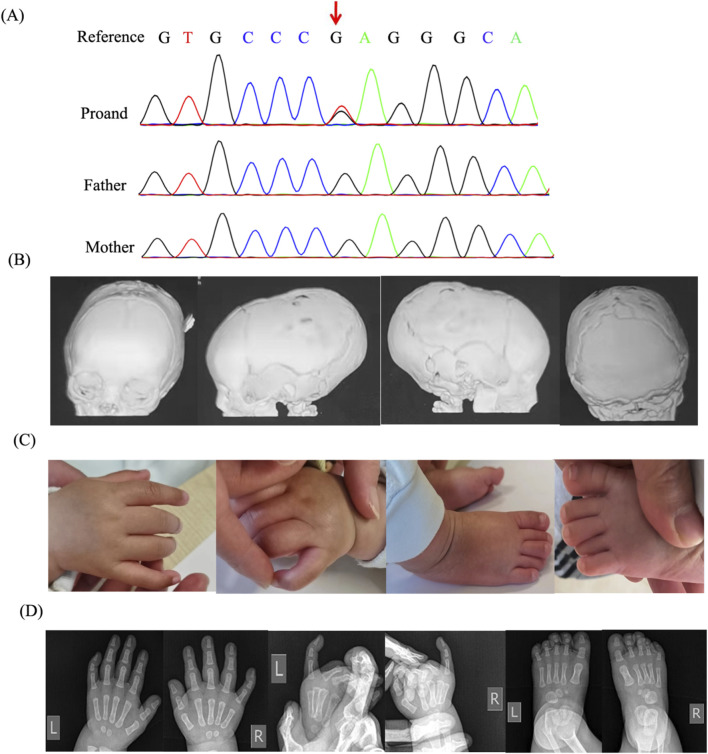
Clinical and genetic features of the proband (P11) harboring the *NOG* variant (c.562G>T, p. Glu188Ter). (A) Sanger sequencing confirming the *de novo* variant. (B) Head CT scan (3D reconstruction, bone window) showing scaphocephaly (elongated anterior-posterior axis), bilateral depression of the cranial vault, and premature fusion of the metopic suture, superior sagittal suture, and right coronal suture. (C) Clinical photograph of the hands demonstrating restricted flexion at the distal interphalangeal joints of the fifth fingers and absence of flexion creases. Clinical photograph of the feet showing upward deviation of the middle toes and absence of creases on toes 2–5. (D) Hand and foot radiographs showing no evidence of bony fusion or synostosis.
